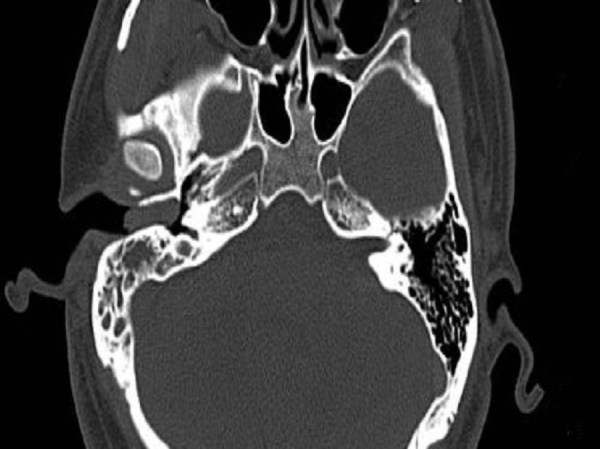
Axial CT scan before surgery showing a well-circumscribed strip of solid mass in the right external auditory canal without bony erosion and a medium-density area in the tympanic antrum.
